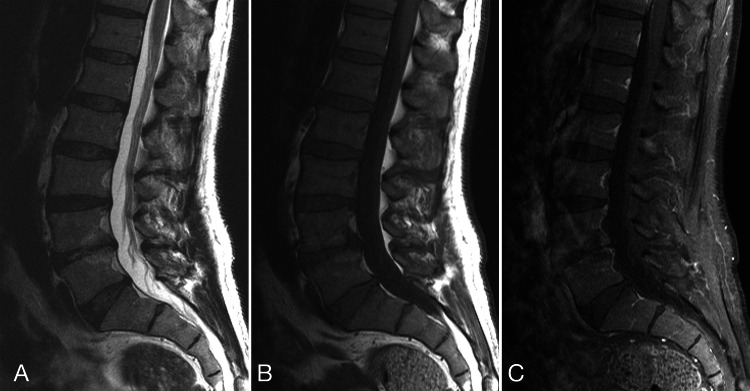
MRI of the lumbar spine. Sagittal T2 (A) and T1-weighted pre-contrast (B) and post-contrast (C) MRI of the lumbar spine. The distal cord, conus, and cauda equina are normal. The spinal canal does not contain a mass or blood products.
